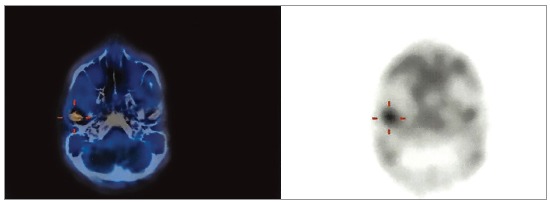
SPECT/CT with hypercaptation of mandibular condyle on the right side.
